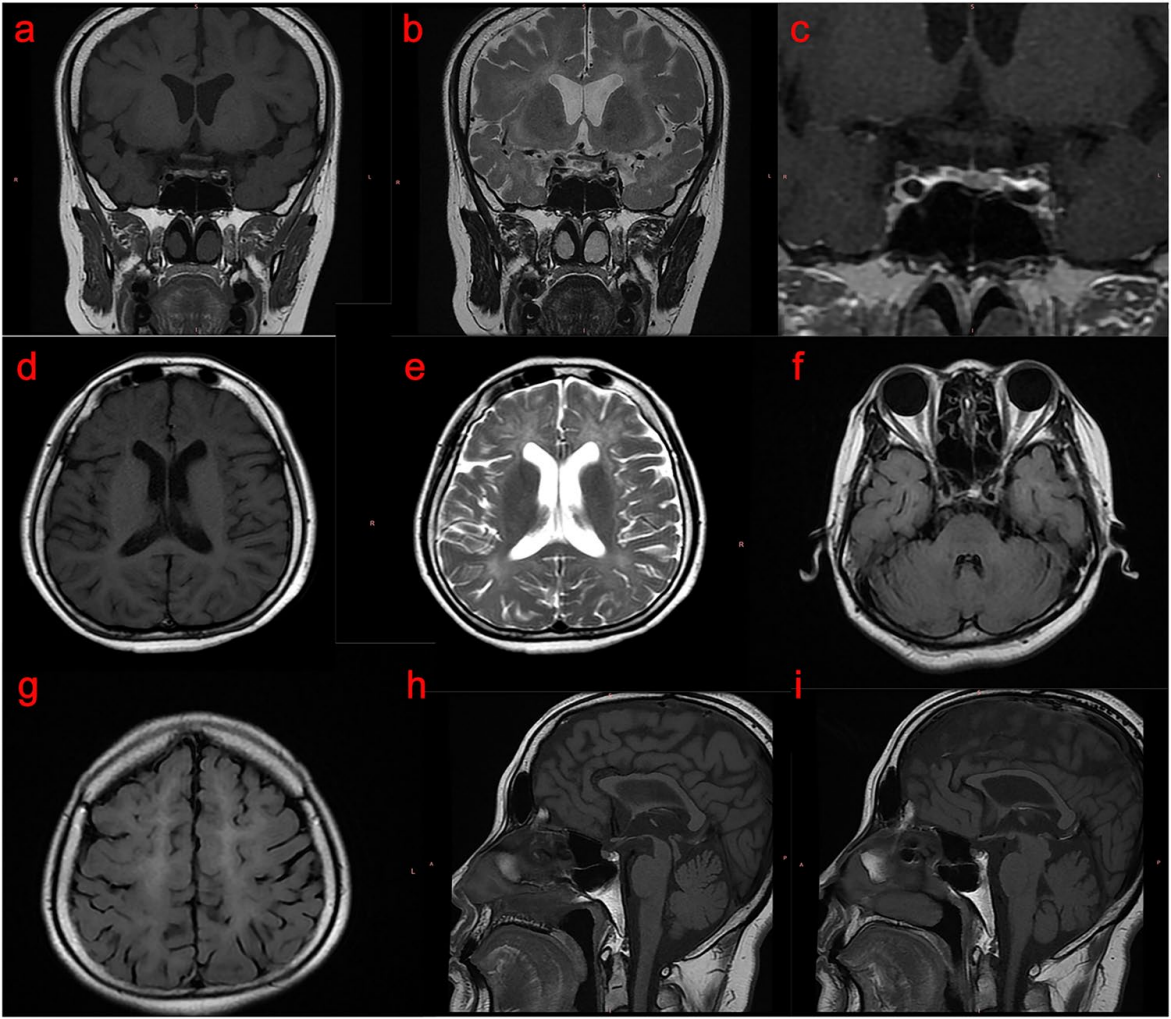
Craniocerebral magnetic resonance imaging (MRI) results of the proband. (a–i) Craniocerebral MRI showed that the pituitary gland was approximately 3.8 mm in height, with a flat upper edge and a slightly sunken saddle base, and multiple tiny nodules with equal and slightly long T1 signals and slightly long T2 signals were seen inside, and the dynamic enhancement showed relatively slightly low signals, the larger one being approximately 2.2 mm in diameter; bilateral periventricular white matter atrophy and degeneration, with short linear and patchy equal and slightly long T1 and slightly long T2 signals, and slightly high T2-Flair signal; cerebral sulcus, cistern, and ventricle were generally widened; corpus callosum atrophy and thinning, particularly at the knee and body of the corpus callosum.
